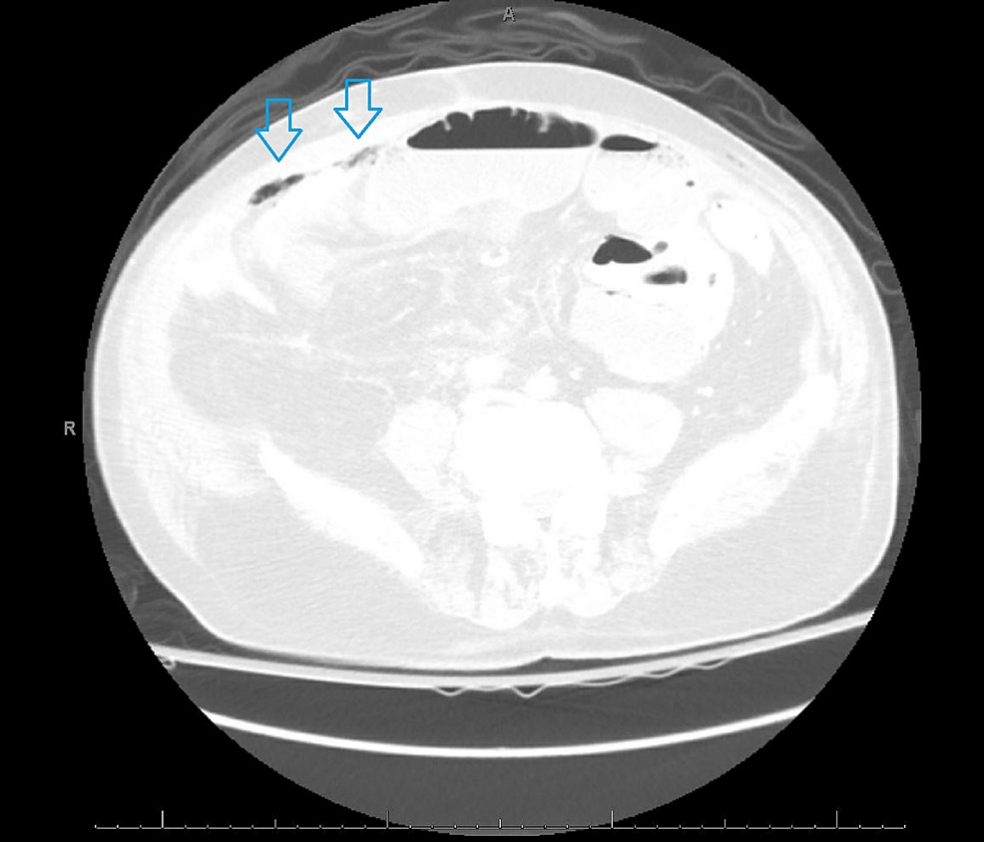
CT scan of the abdomen, lung window level. Blue arrow: Pockets of free air seen on lung window.
